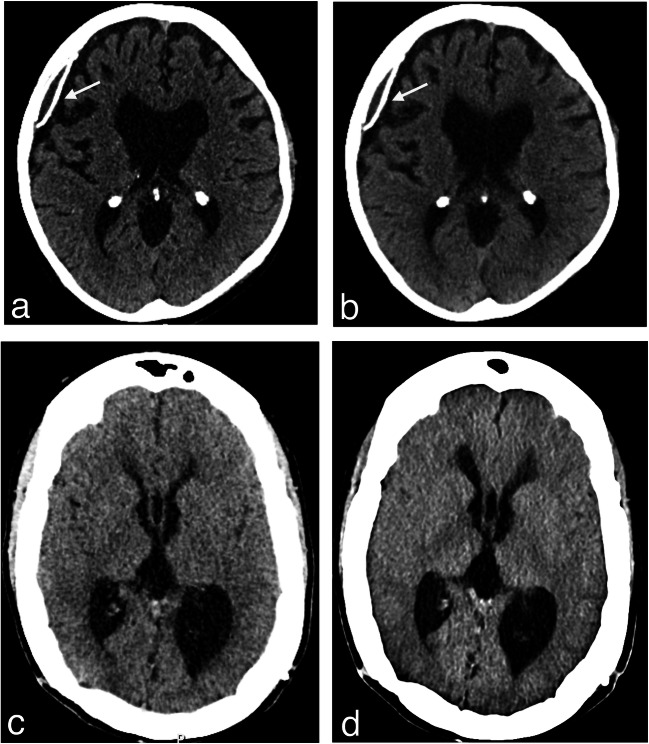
Stability of ventricular system after VAS inactivation. CT scans obtained from patient 1 (a and b) and patient 2 (c and d) before surgery (a and c) and after removal of the VAS catheter from the atrium and definitive inactivation of the shunts (b and d): no appreciable increase in the diameter of the cyst and ventricles is visible. Arrows in Fig. 4a and b point to the calcified wall of the cyst
